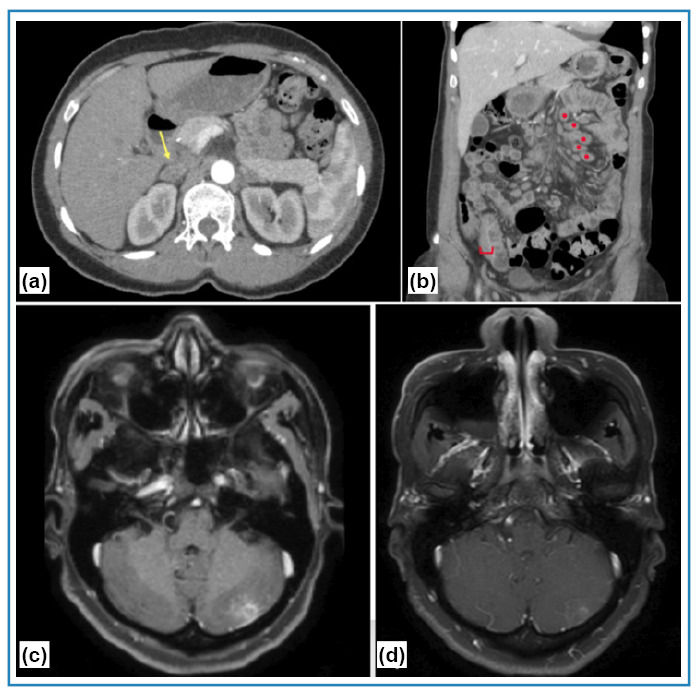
Abdominal Computed Tomography: (a) Diffuse adrenal enlargement (yellow arrow); (b) Significant parietal thickening of the distal ileum (red bracket) and multiple enlarged lymph nodes in mesenteric chains (red dots). Brain magnetic resonance imaging (MRI): (c) Peripheral oval focal lesion in the left cerebellar hemisphere, with ring enhancement by gadolinium, associated with adjacent leptomeningeal impregnation; (d) Regression of the oval focal lesion in the left cerebellar hemisphere (day 40 of treatment).
